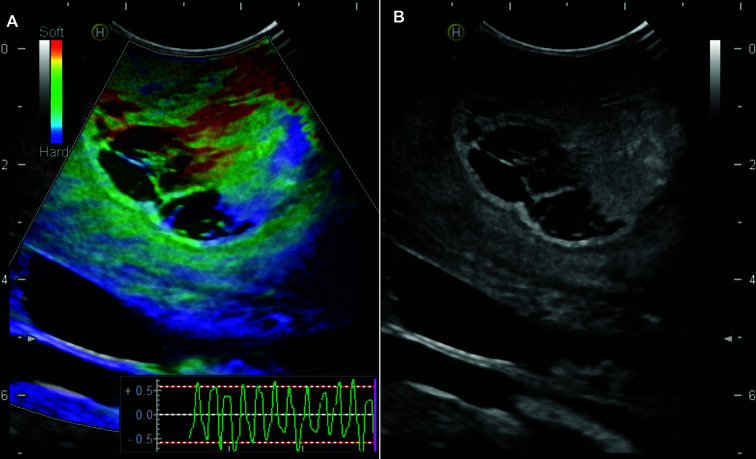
Example of intraoperative ultrasound images. 65-year-old man with a right frontal glioblastoma. (A) Elastogram showing the difference in consistency between the tumor and the peritumoral region (green - red) from the rest of the healthy parenchyma (blue). In the right-lower part of the image, a graphic representation of the external compression waves is observed. (B) Simultaneous image in B-mode.
